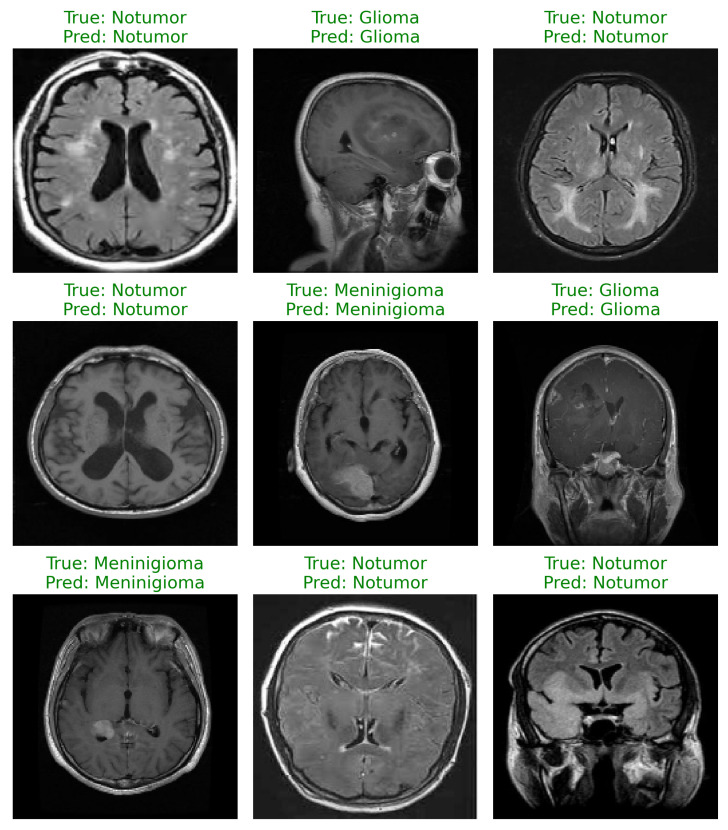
Qualitative visualization of Custom CNN classification results for brain MRI images across four categories: Glioma, Meningioma, Pituitary, and No Tumor.
